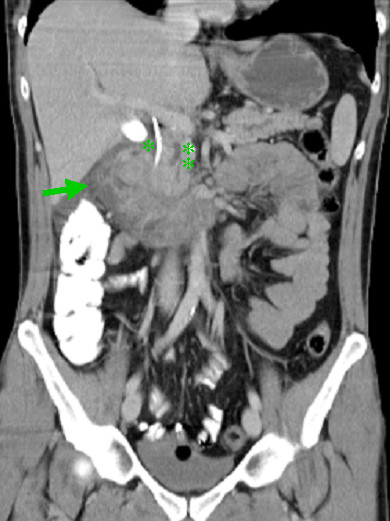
Adenomyoma of the pancreas: abdominal computed tomography scan reveals severe duodenitis (↦), a stent in the common hepatic duct (*) and a dilated duct of Wirsung ().
